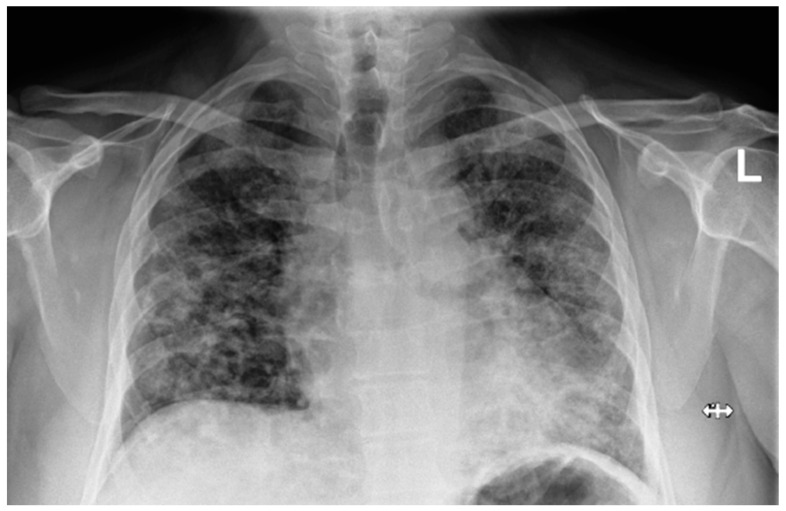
Chest X-ray at the follow up: patient 5. Partial regression of bilateral lung infiltrates (the “L” orientation marker indicates the left side).
